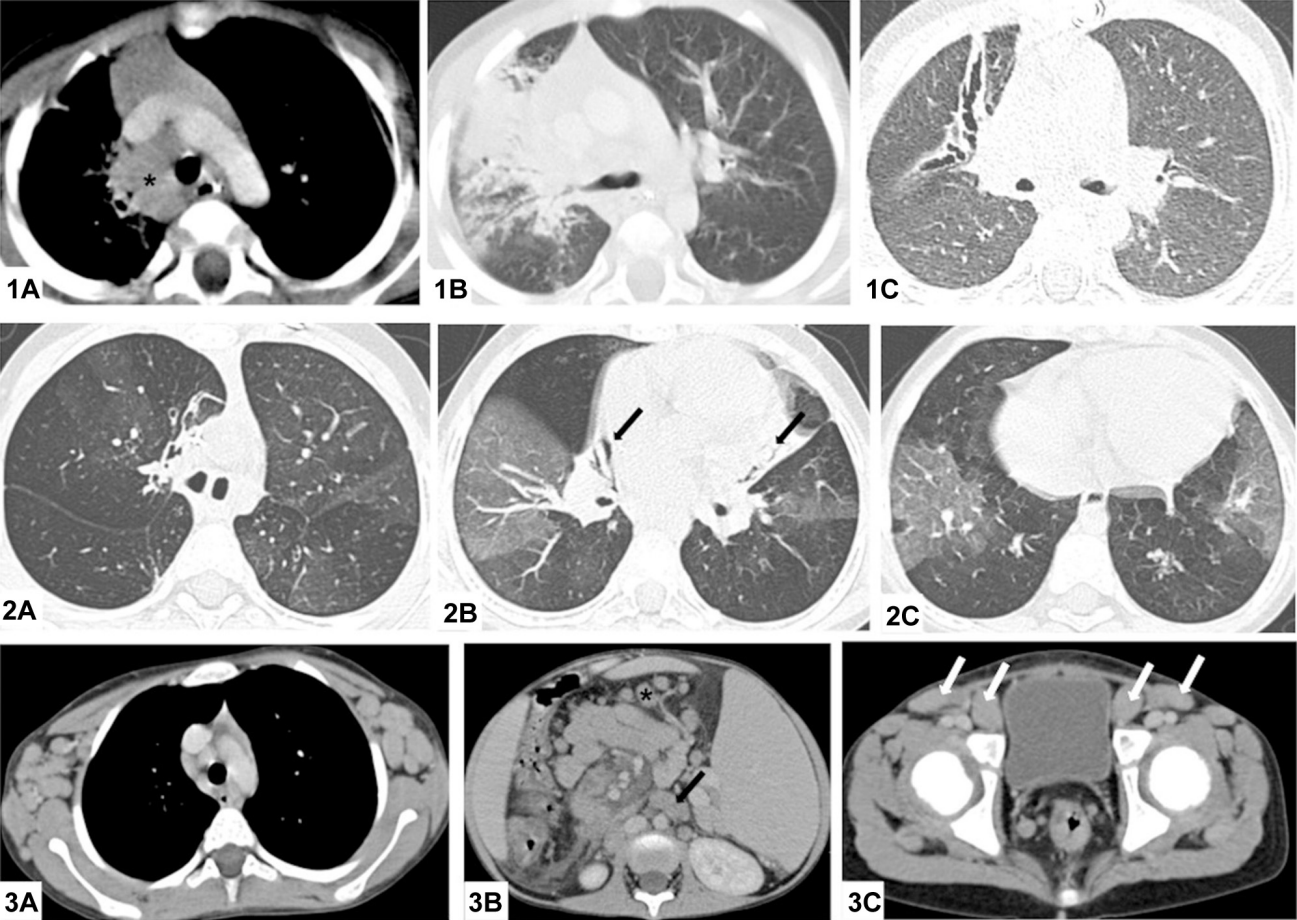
Radiology of patients with APDS. *1*, CT scan of the chest (2-year-old boy), demonstrating right paratracheal lymphadenopathy *(A)*, right upper lobe consolidation, and centrilobular nodules *(B)*, progressing 2 years later to severe right upper lobe bronchiectasis *(C)*. *2*, CT scan of the chest *(A-C)* in a 7-year-old boy reveals widespread mosaic attenuation (indicative of small airways disease), mild right upper lobe bronchiectasis *(A)* and atelectasis (*B*, *black arrows*). *3*, CT scan of the chest *(A)*, abdomen *(B)*, and pelvis *(C)* of an 8-year-old boy showing axillary, paratracheal, para-aortic *(black arrow)*, mesenteric and inguinal lymphadenopathy *(white arrows)*, and splenomegaly.
